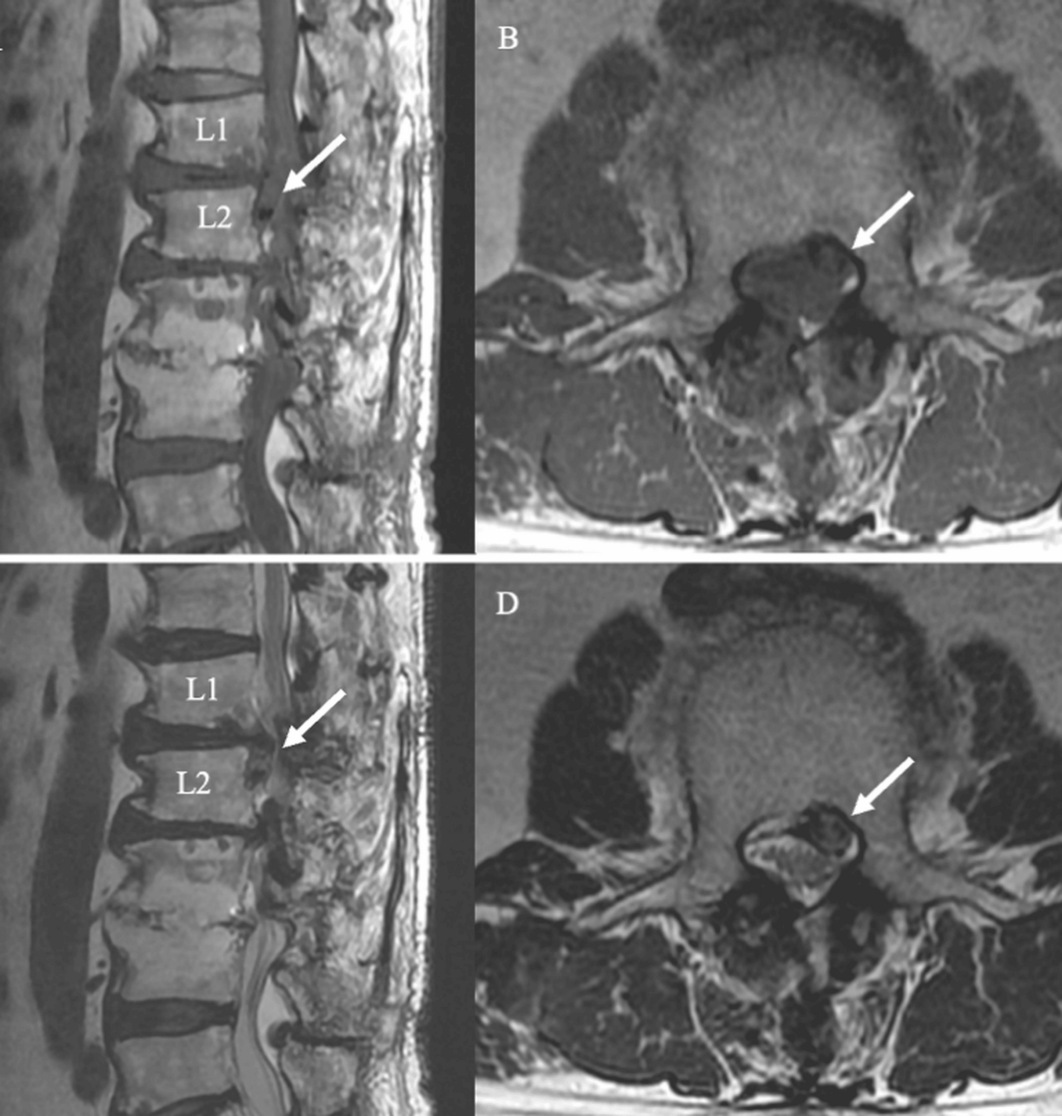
Initial MRI Findings of L1/2 Disc Herniation Sagittal and axial T1-weighted (A, B) and T2-weighted (C, D) MRI scans obtained 10 months before surgery show a small gas-containing disc herniation at the L1/2 level *(white arrow)*. The lesion appears as low signal intensity and mildly compresses the dural sac.
